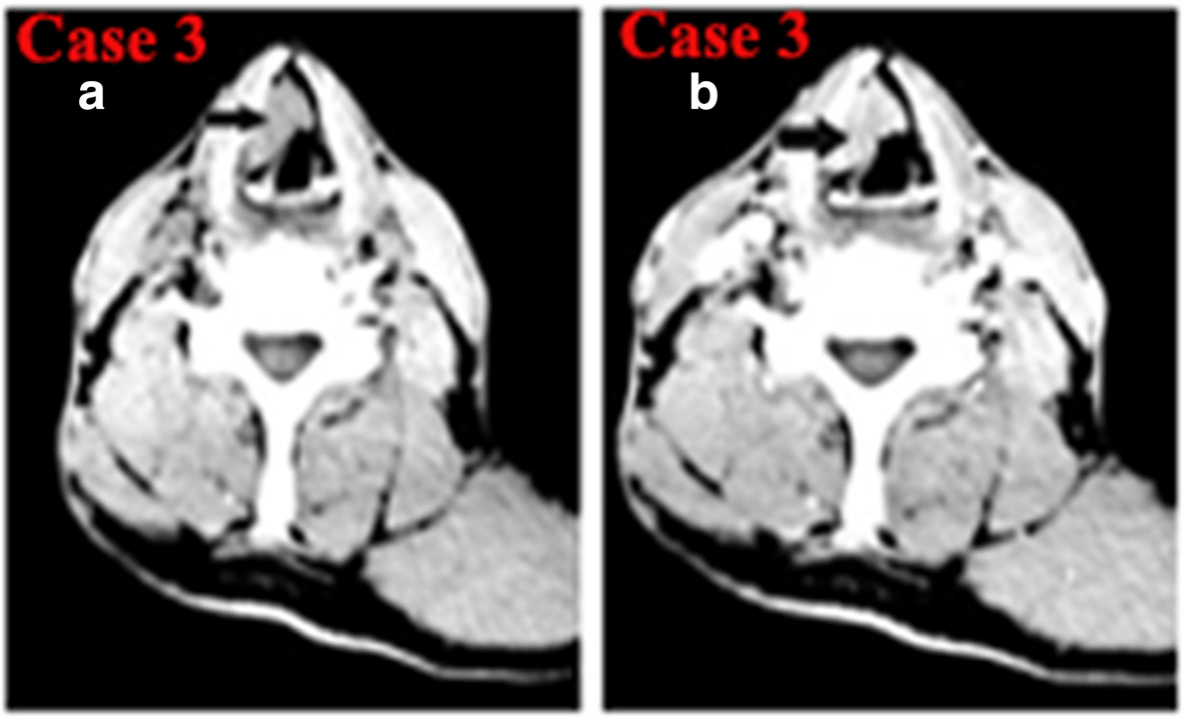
CT of laryngeal small-cell neuroendocrine carcinoma of Case 3. a) Noncontrast axial CT showing a heterogeneous irregular soft-tissue mass in the right supraglottic region involving the right vocal cord. b) Contrast-enhanced CT showingthat the lesion was heterogeneous enhanced and the thyroid cartilage was not involved.
